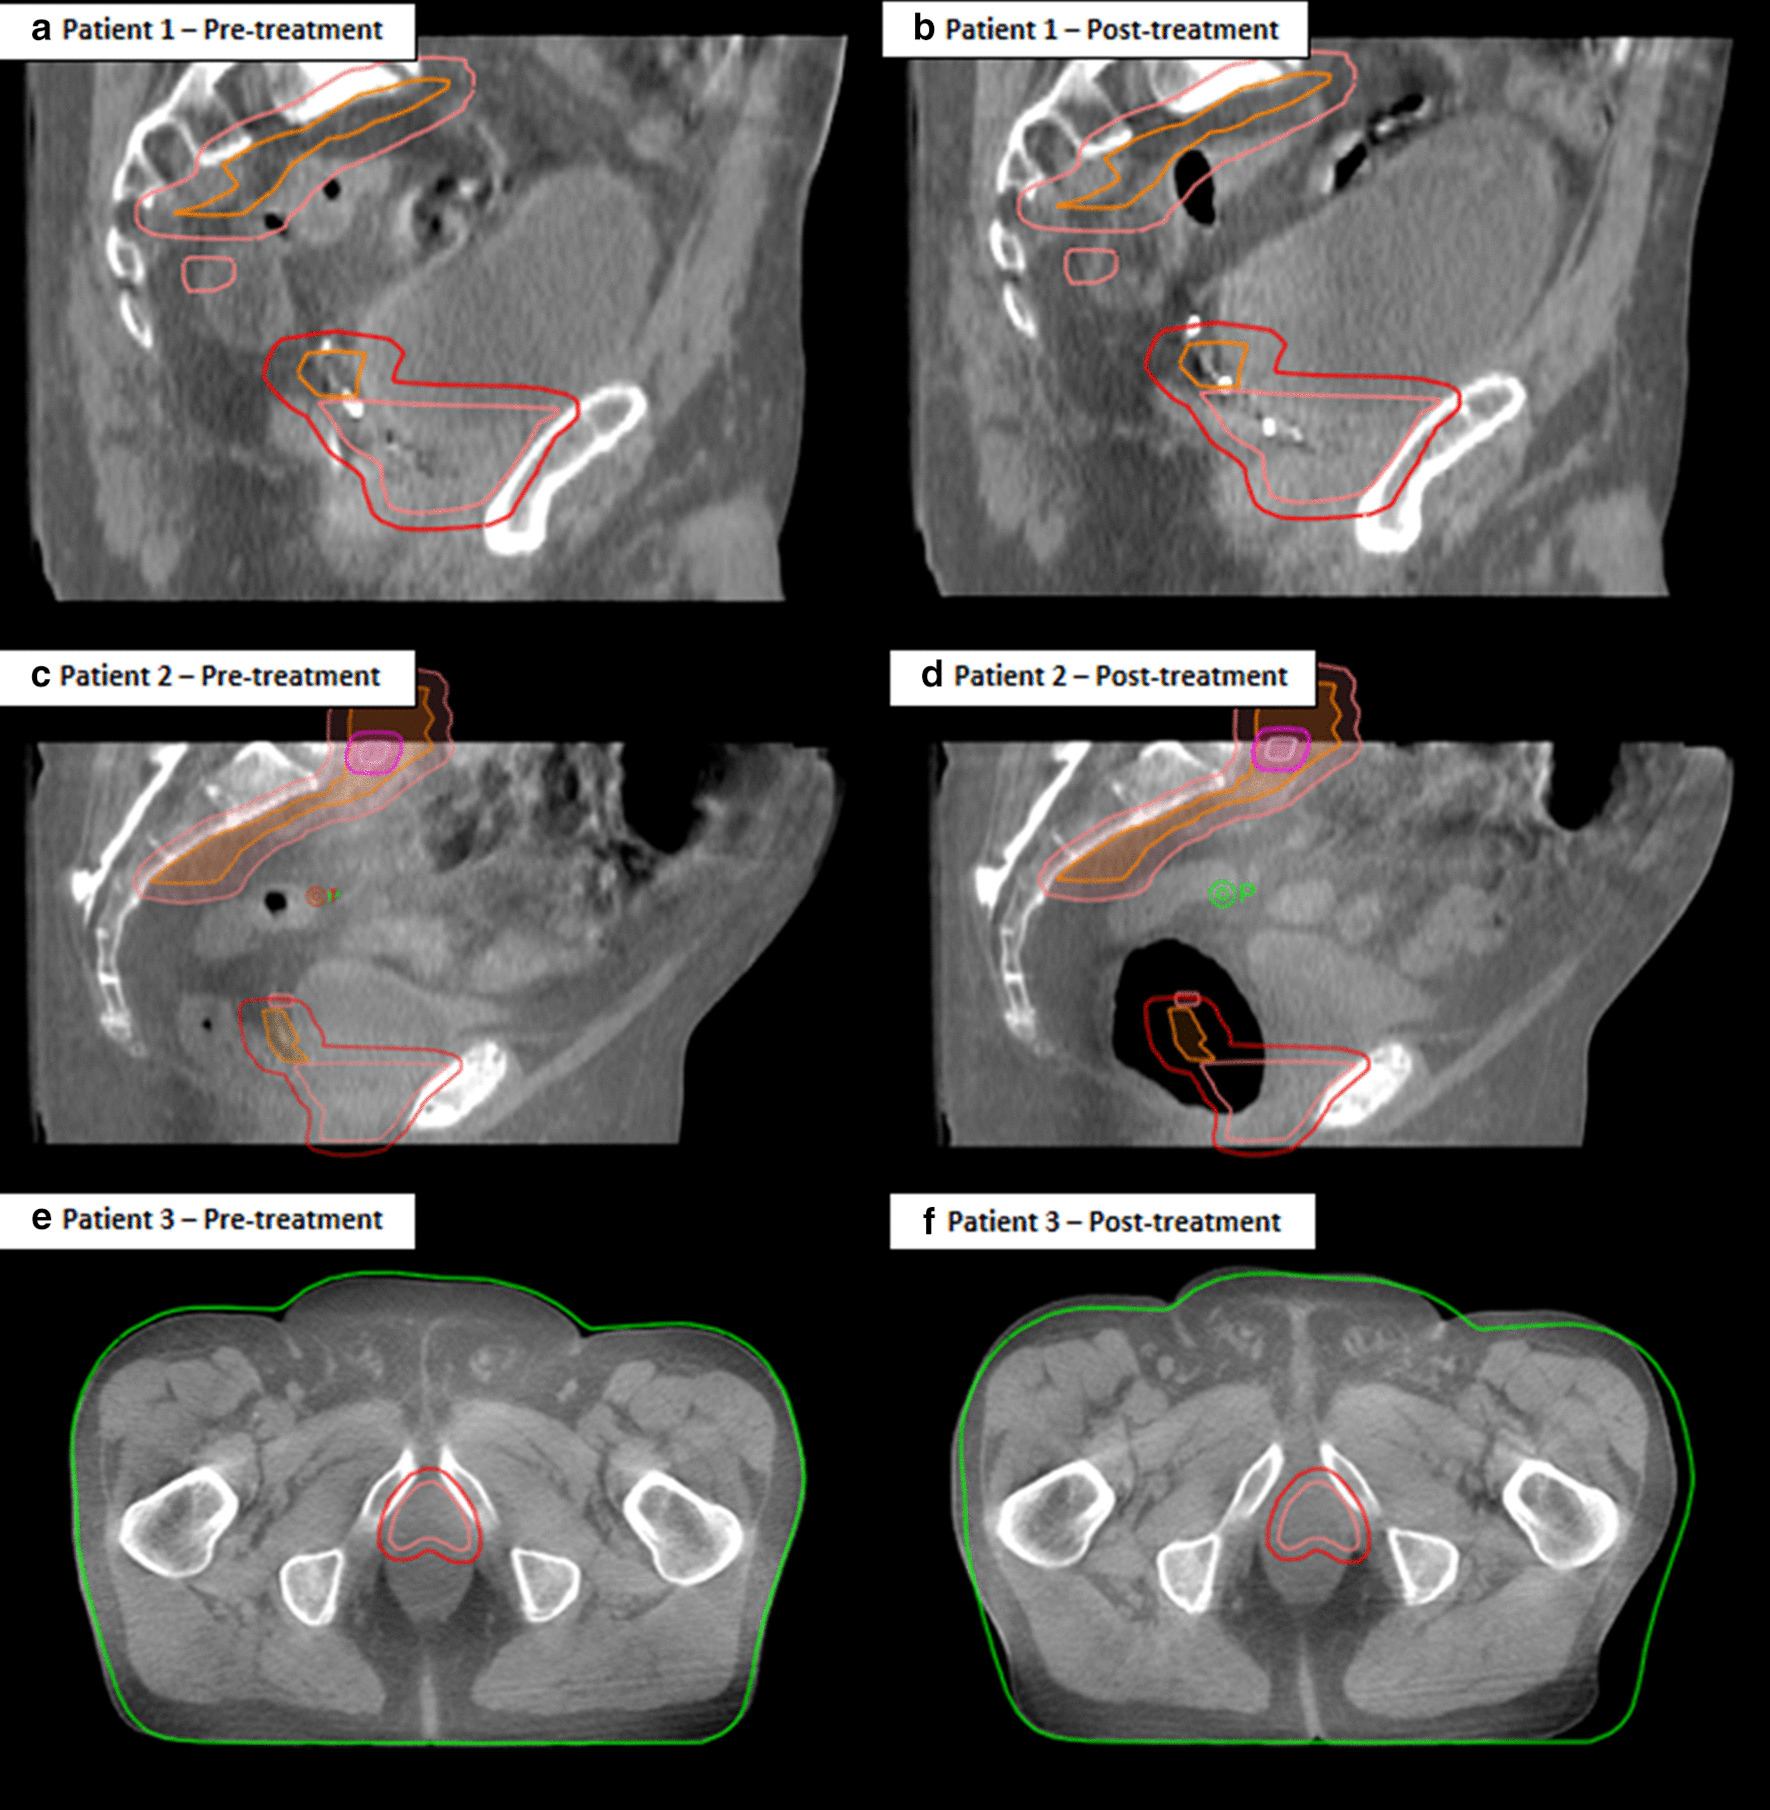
Images showing intra-fraction displacement. The pre (a, c, e) and post-treatment (b, d, f) CBCT scans for 3 patients are displayed. In patient 1′s pre-treatment a the superior clip in within the prostate bed PTV (red volume) but on the post-treatment b the bladder had continued to fill by a small amount and caused the prostate bed and superior clip to move posterior and superior causing a marginal miss. Patient 2’s pre-treatment c had little rectal gas present but on the post-treatment d a large amount of gas appeared in the prostate bed region which caused the prostate bed to move anteriorly resulting in a marginal miss. The third patient’s pre-treatment e showed relaxed buttock check and in the post-treatment f the left buttocks cheek had tensed after the bladder continued to fill and caused the prostate bed to move laterally
